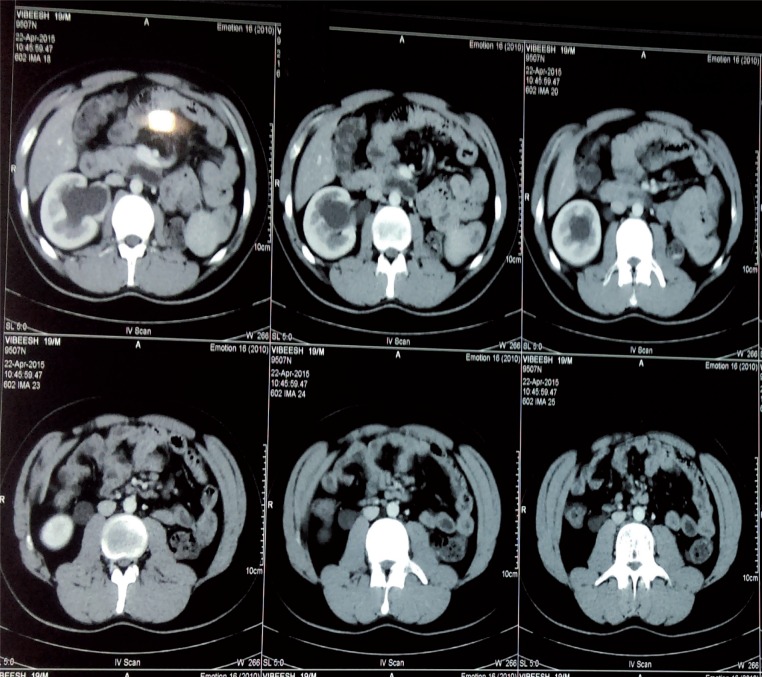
CT scan image, shows absent left kidney and right hydronephrosis and hydroureter up to L3 vertebra at which level it passes behind IVC.
